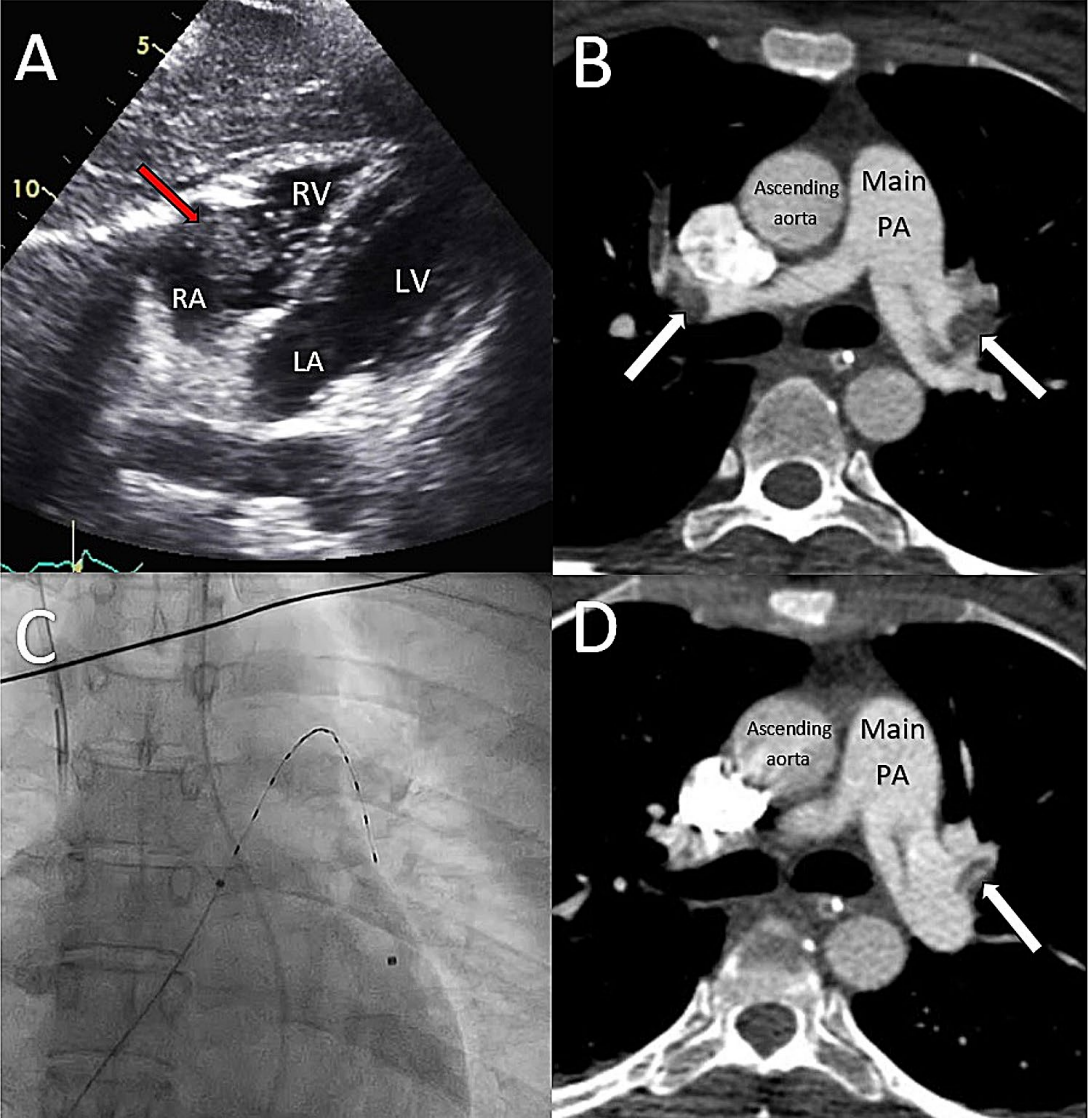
a Transthoracic echocardiogram from the subcostal view showing large RA thrombus, b CTPA showing bilateral PE, c EKOS catheter in left PA, d repeat CTPA 3 days later showing reduced clot burden in left PA
